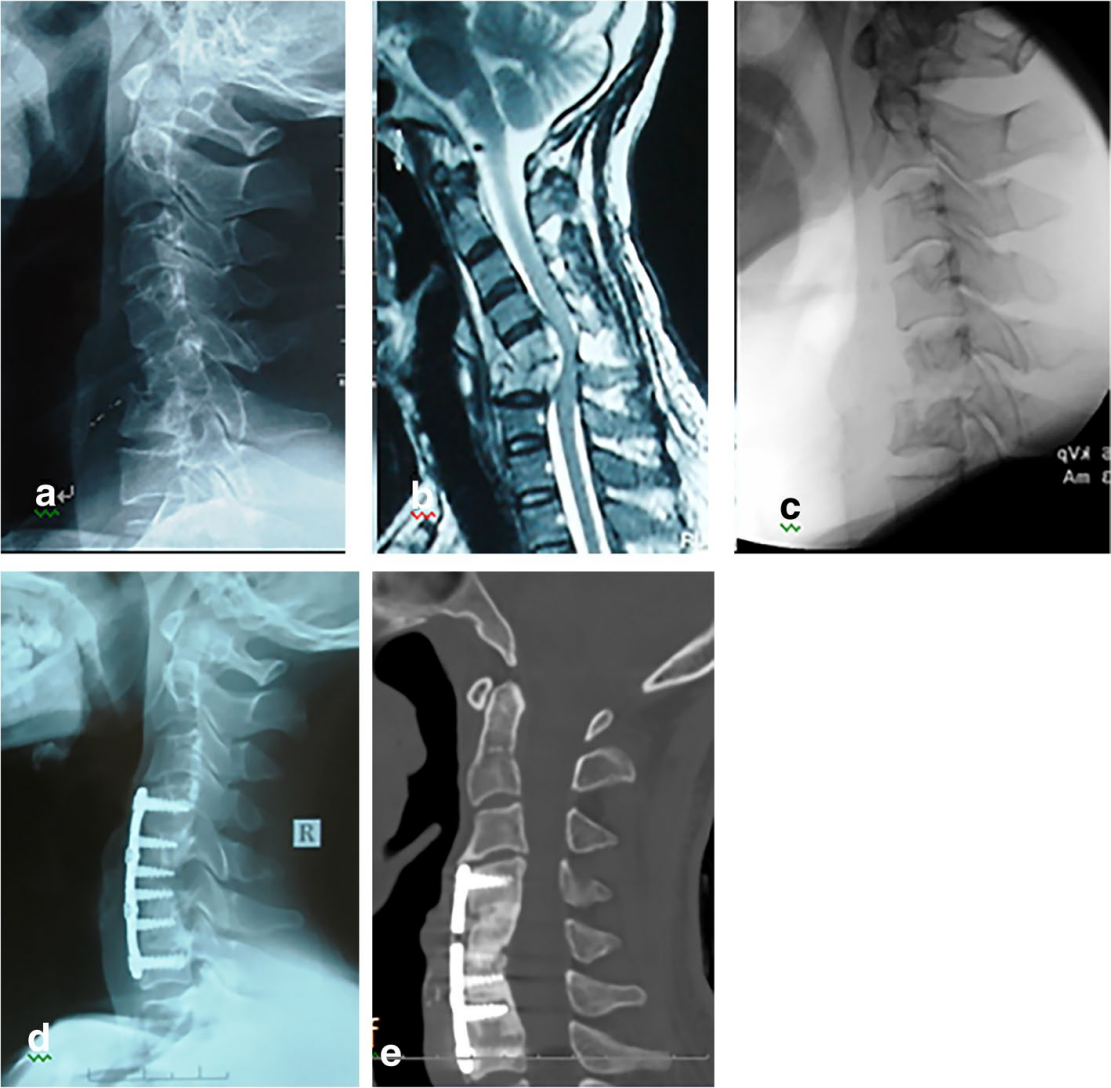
A 46-year-old male patient with cervical spine tuberculosis (C5–6). a-c Preoperative radiography and MRI showed destruction of C5, C6 vertebrae with kyphosis and spinal cord compression. c X-ray showed reduction of cervical kyphosis after intraoperative traction. d X-ray demonstrated anterior debridement, iliac bone grafting and internal fixation from C4 to C7. e CT scan at 12 months of follow-up showed bone fusion at the interface
